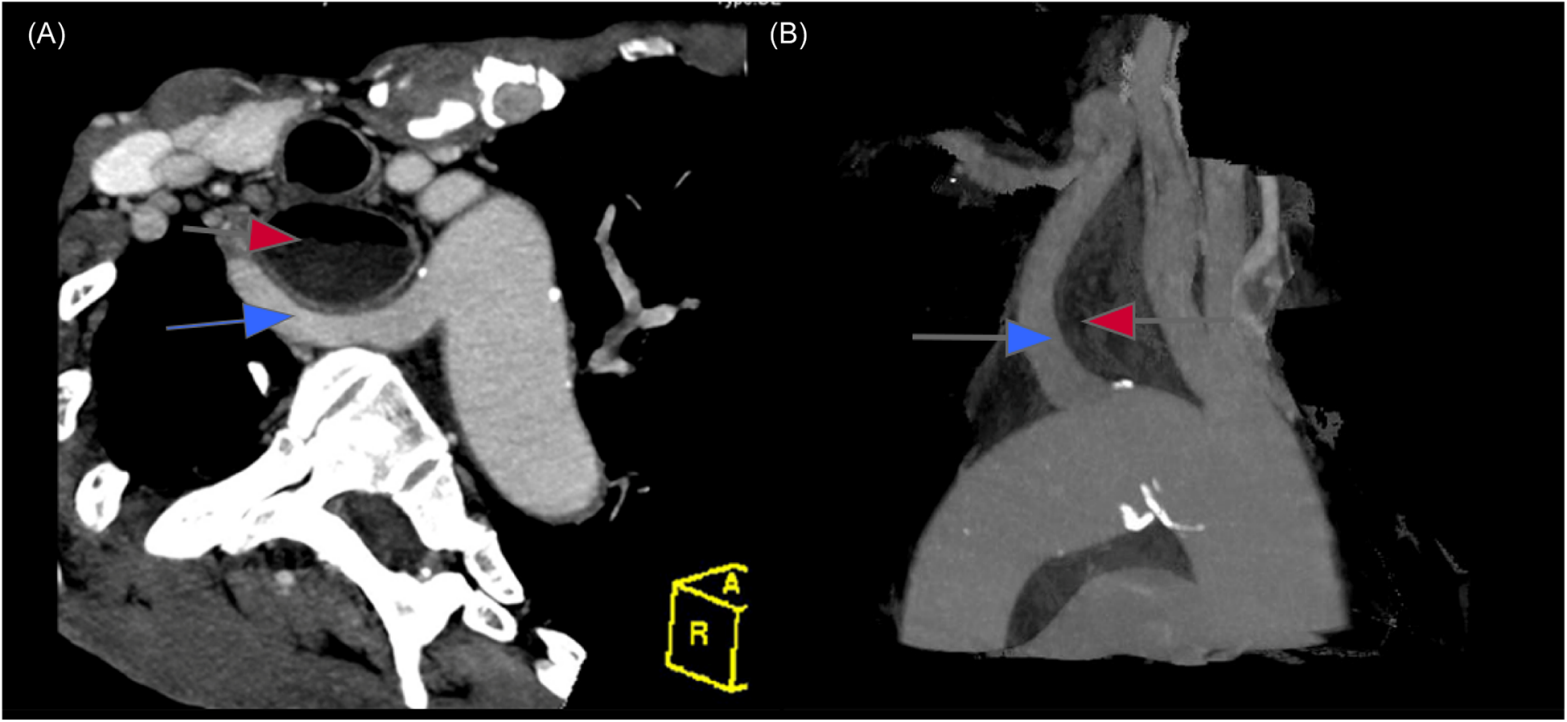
Computed tomography (axial reformation (A) and sagittal reformation (B) showed an ARSA (blue arrow) extends from the left side of the aortic arch and running bhind the esophagus (red arrow).
